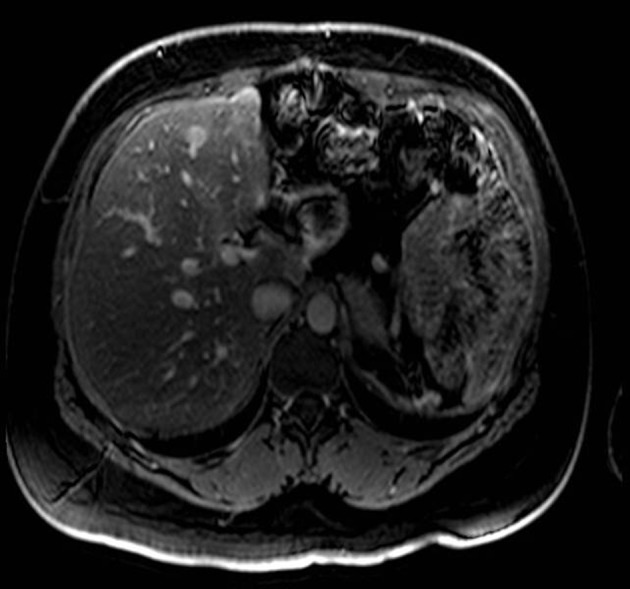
MRI with gadolinium contrast demonstrating a metastases in segment 4 of the liver.
